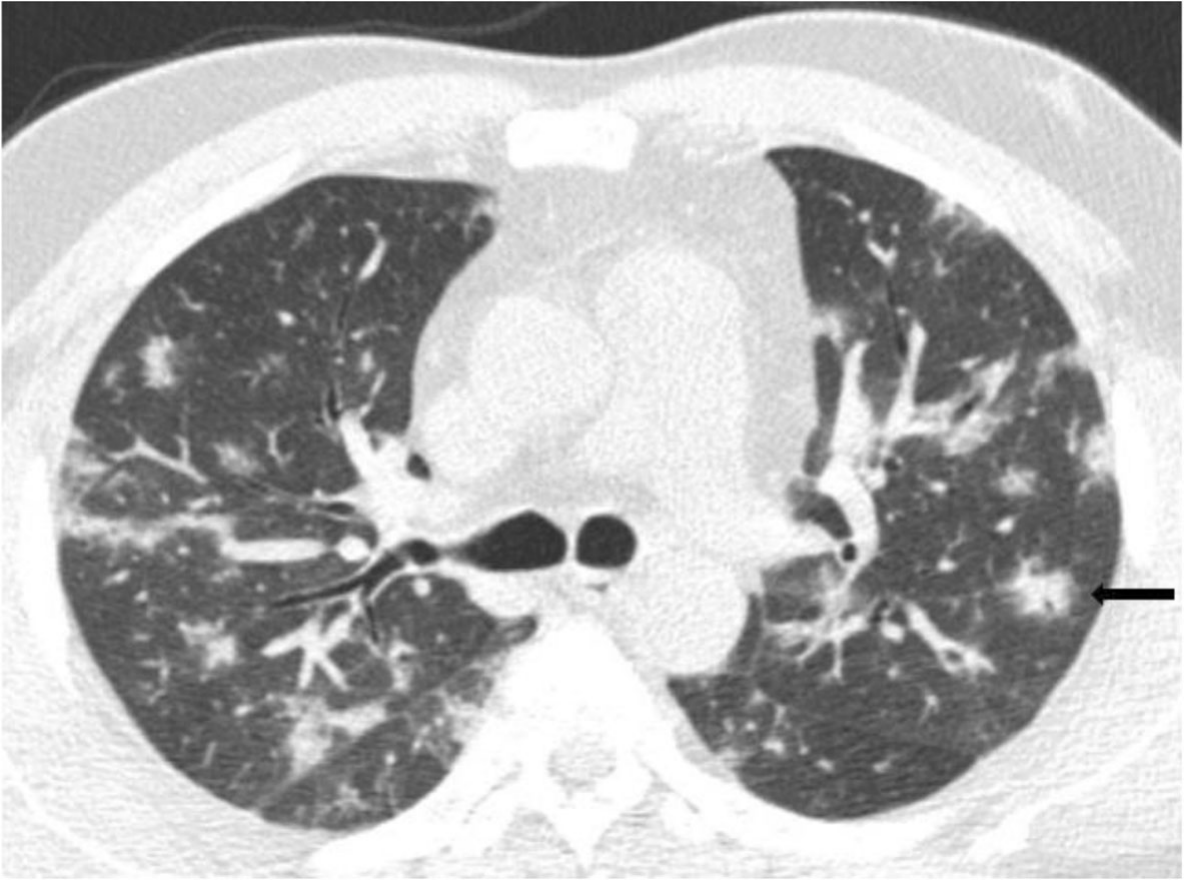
Halo sign. Axial CT thorax of a 40-year-old COVID pneumonia patient showing multiple bilateral patchy consolidations with surrounding ground-glass opacities as indicated by the arrow
